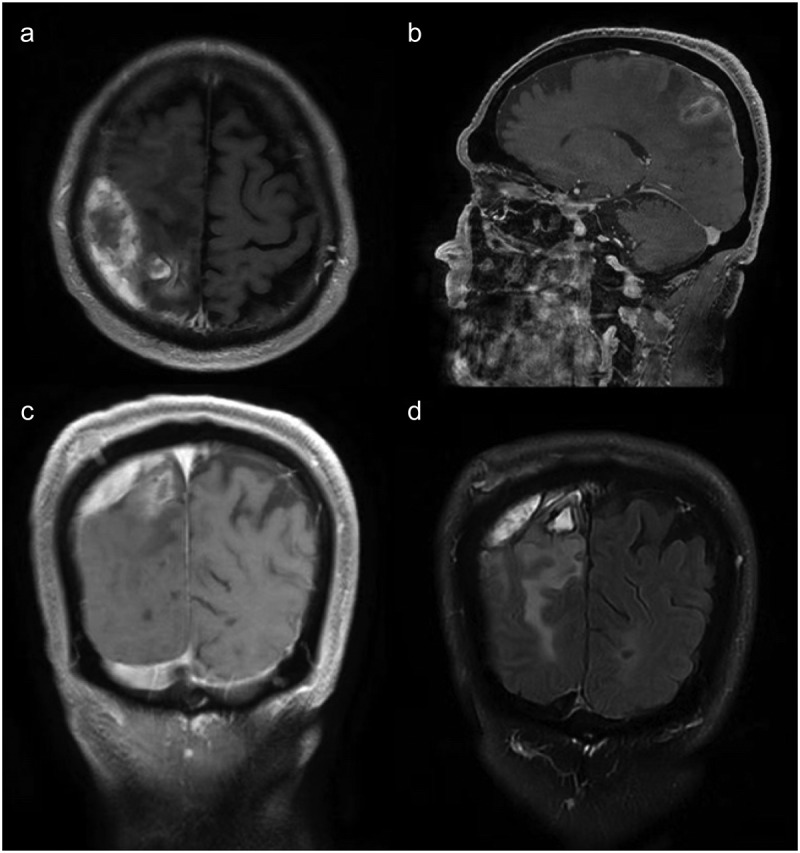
MRI of the brain before discharge (A–D) Showed that the pus cavity in the right parietal lobe had notably narrowed, and peripheral cerebral edema had slightly reduced compared to previous imaging.
